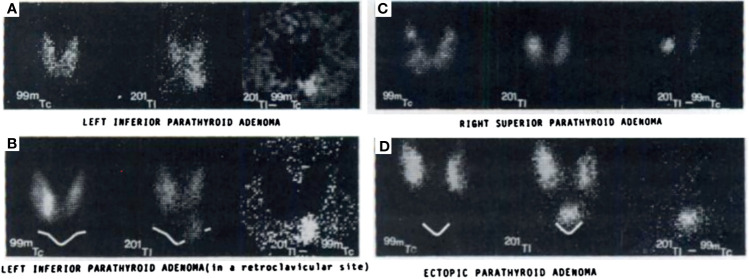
Early examples of parathyroid pathology detection using ^99m^Tc-pertechnetate and thallium subtraction scintigraphy. Upper left panel (A) depicts ^99m^Tc-pertechnetate thyroid uptake on the left image, ^201^Tl uptake in the middle image, and the subtraction image highlighting a left inferior parathyroid adenoma on the right image. Lower left panel (B) depicts a similar ^201^Tl minus ^99m^Tc-pertechnetate series of three images of a left inferior parathyroid adenoma with decreased signal to noise ratio due to its location behind the clavicle contributing to increased attenuation relative to the series above. Right upper panel (C) depicts a similar subtraction series of a right superior parathyroid adenoma. Right lower panel (D) depicts a subtraction series of an ectopic parathyroid adenoma near the sternal notch. Reproduced with permission from: *Ferlin G, Borsato N, Camerani M, Conte N, Zotti D. New perspectives in localizing enlarged parathyroids by technetium-thallium subtraction scan. J Nucl Med. 1983 May;24(5):438-41. PMID: 6842292*.
